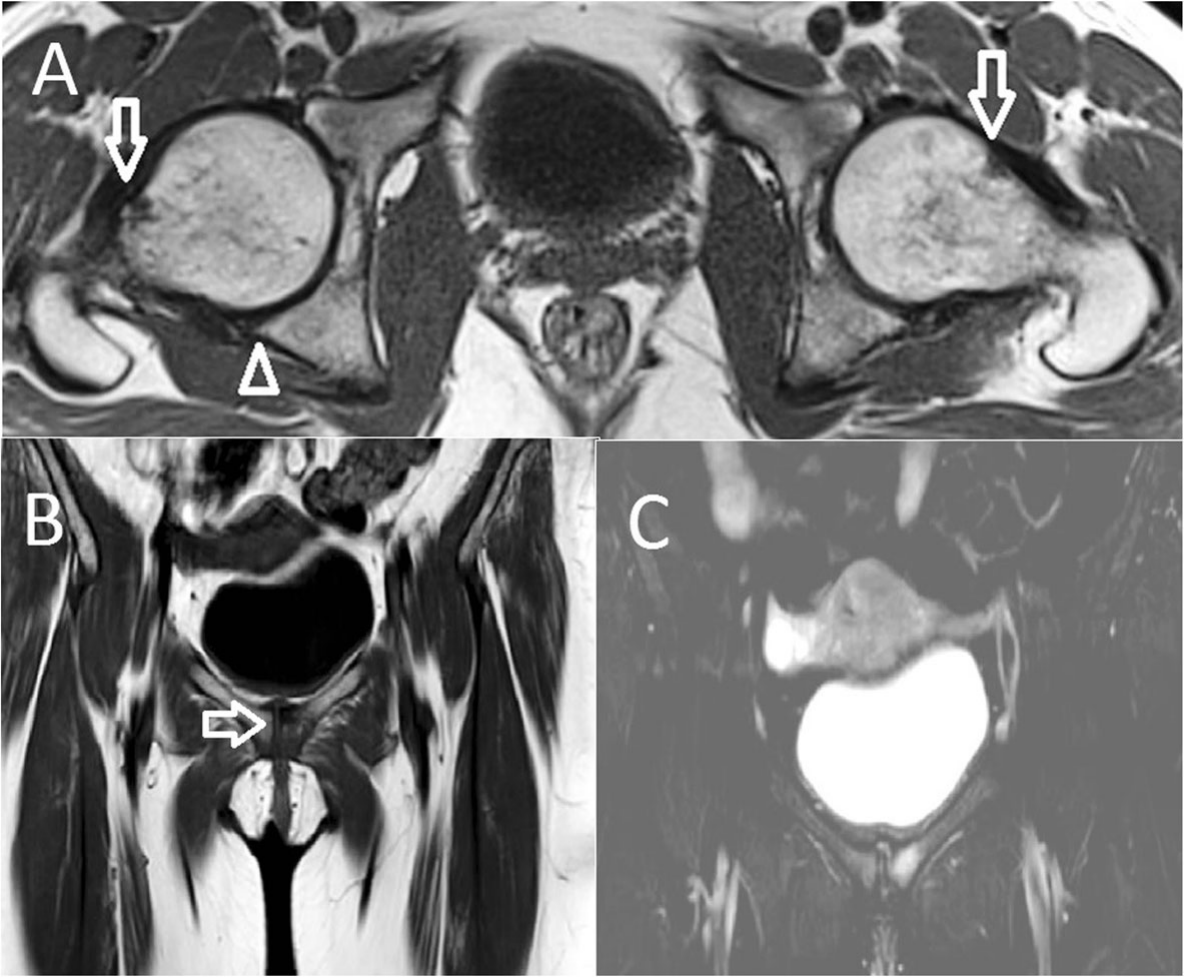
Fifty-year-old female who had a 3-month history of pubic pain with diagnosed femoroacetabular impingement. a Axial T1-weighted MR image shows bilateral osseous bumps at the femoral head and neck junctions (thin arrows) and bilateral synovial herniation pits on the left and right side (arrow) and minimal osteophytes (arrowheads). b Coronal T1-weighted MR image with osseous irregularity on both sides of the symphysis pubis (arrow). c Coronal fat-saturated T2-weighted MR image shows mild osteitis pubis with left-side predominant subchondral bone marrow edema
